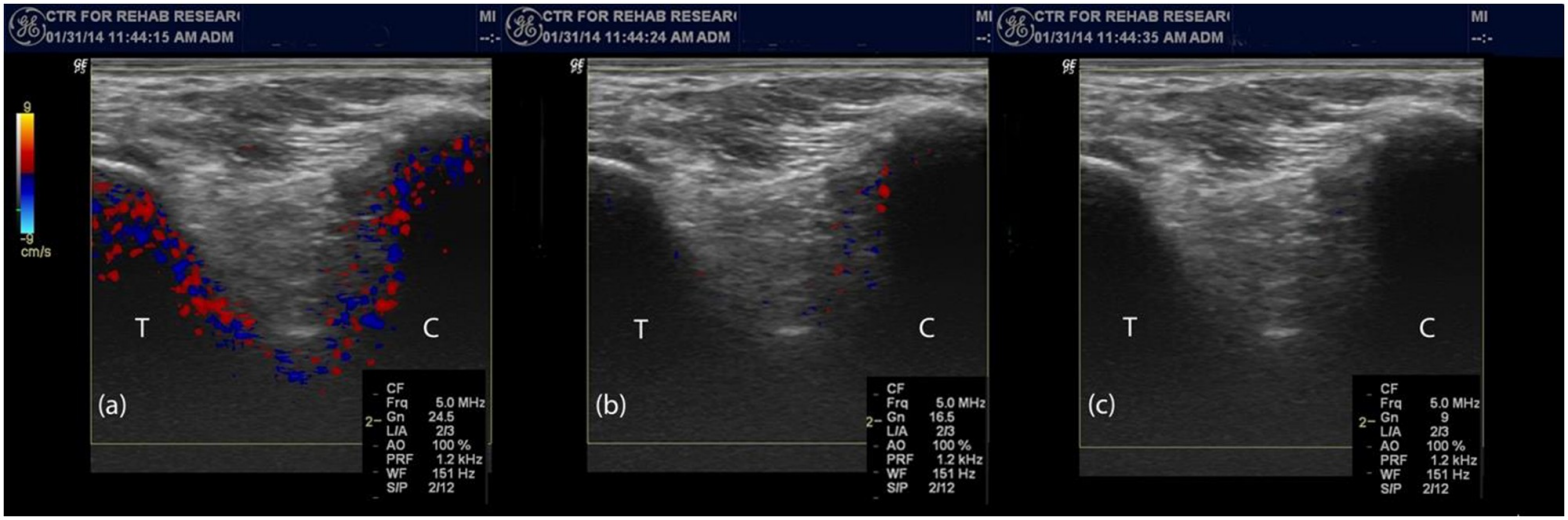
Color Doppler images of the STJ from the lateral side in three different levels of Threshold Unit (TU) settings. (a) with TU setting high, vibration detected on both talus and calcaneus as recognizable by color pixels on both talus and calcaneus; (b) vibration no longer detected in talus = TU_talus_; as recognizable by missing color pixels on talus; (c) vibration no longer detected in calcaneus = TU_calcaneus_: as recognizable by missing color pixels on calcaneus: T = talus and C = calcaneus.
